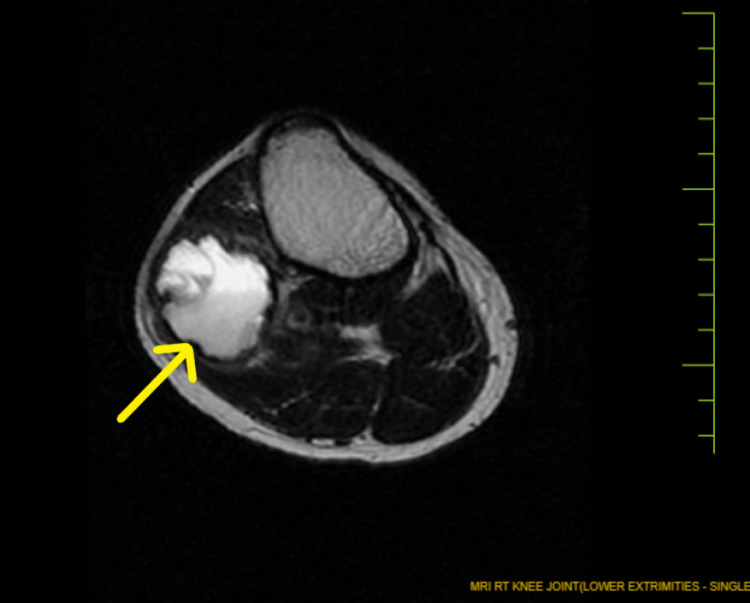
Transverse section of the magnetic resonance imaging of the right knee joint showing the cystic lesion marked by the arrow and the blood fluid level.
